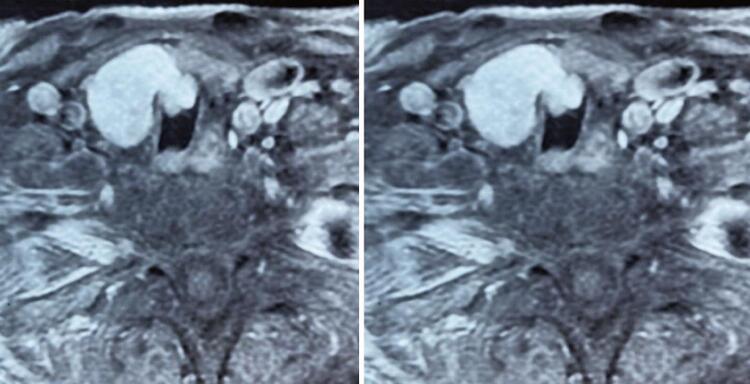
Contrast-enhanced magnetic resonance imaging showing cervical tumor extending to the tracheal lumen
